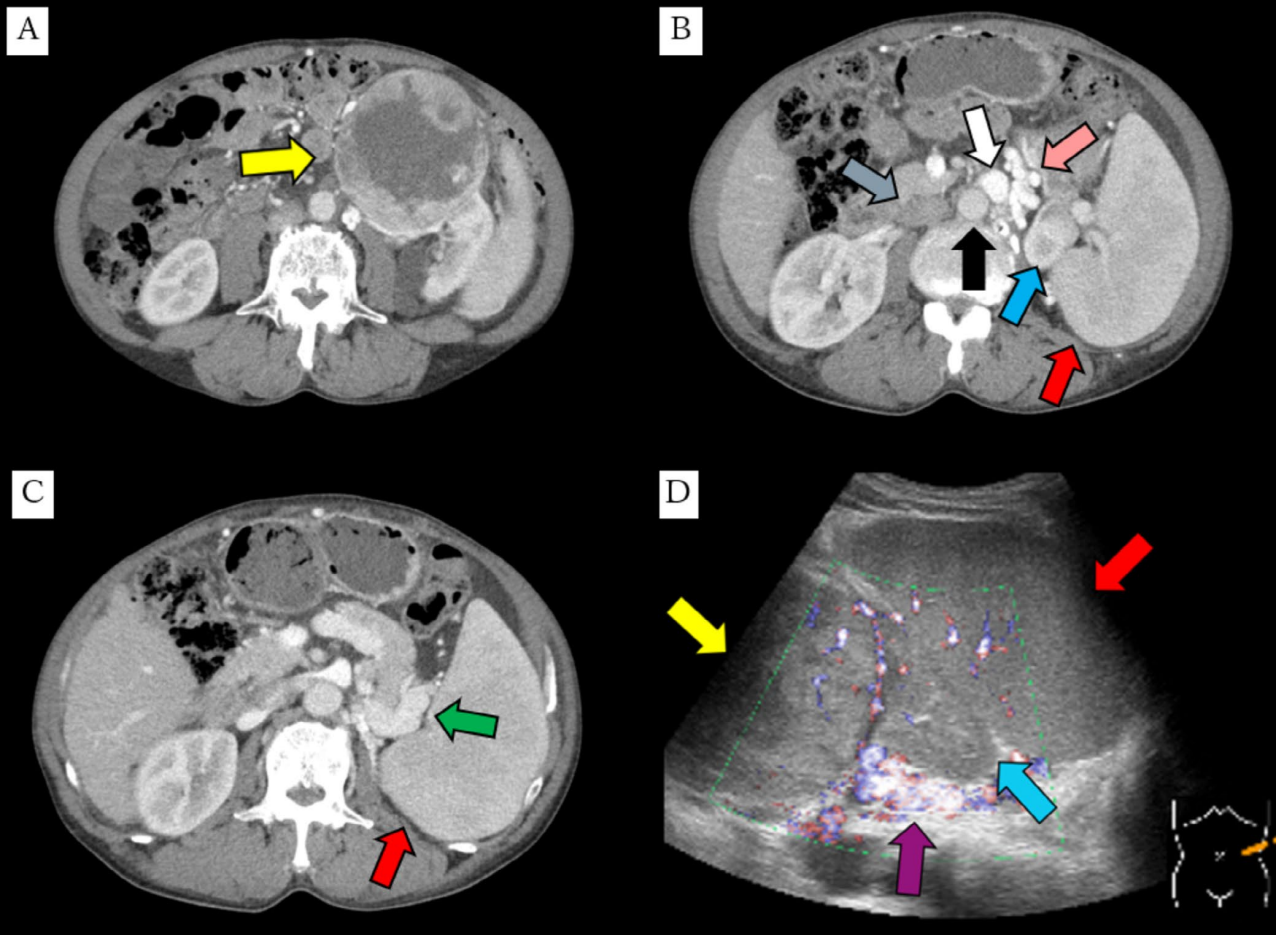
Contrast‐enhanced CT of the abdomen revealed a 10‐cm renal tumor (A) and well‐developed collateral blood vessels (pink arrow), enlarged spleen (red arrow), normal kidney (blue arrow), aorta (black arrow), inferior vena cava (gray arrow), renal vein (B), and dilated and tortuous splenic vein (green arrow) (C). Abdominal ultrasound showed a renal tumor (yellow arrow), normal kidney (blue arrow), enlarged spleen (red arrow), and splenorenal shunt (purple arrow) (D).
